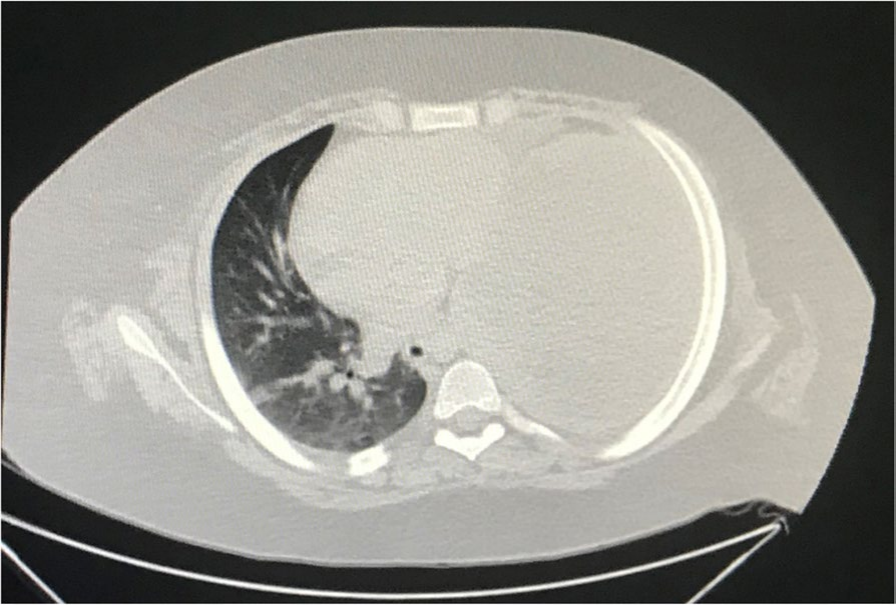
Emergency Chest High resolution computed tomography scan cut, showing severe empyema and complete collapse of the left lung in a 12-year-old patient with coronavirus disease
